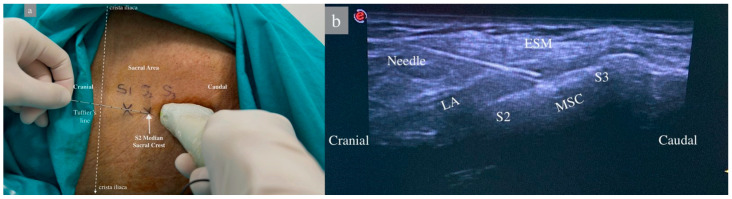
Patient position and sonoanatomy during ultrasound-guided sacral erector spinae plane block. (a) Patient in lateral decubitis position. The dashed white lines represent Truffier’s line, a transverse line connecting the upper parts of the crista iliacae. S1–2–3: Level of 1st, 2nd, and 3rd sacral vertebrae median crests. (b) ESM: Erector Spinae Muscles, LA: Local Anesthetic, MSC: Median Sacral Crest, S2–S3: Level of sacral vertebrae.
